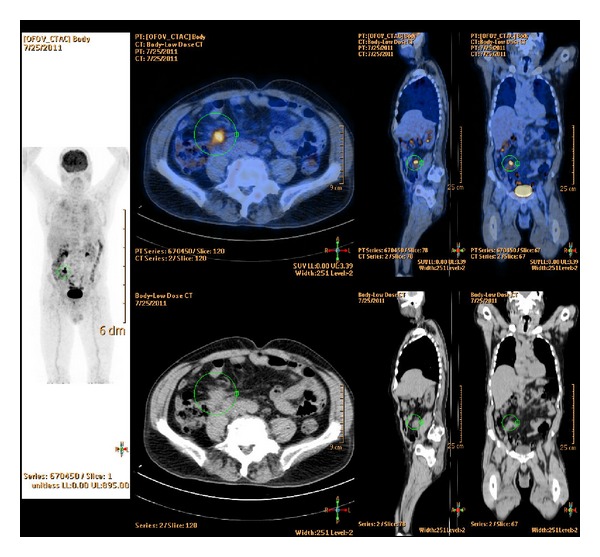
F-18 FDG PET/CT showing enhancement and intense abnormal tracer uptake focus in the appendiceal nodule in front of the right psoas major.
